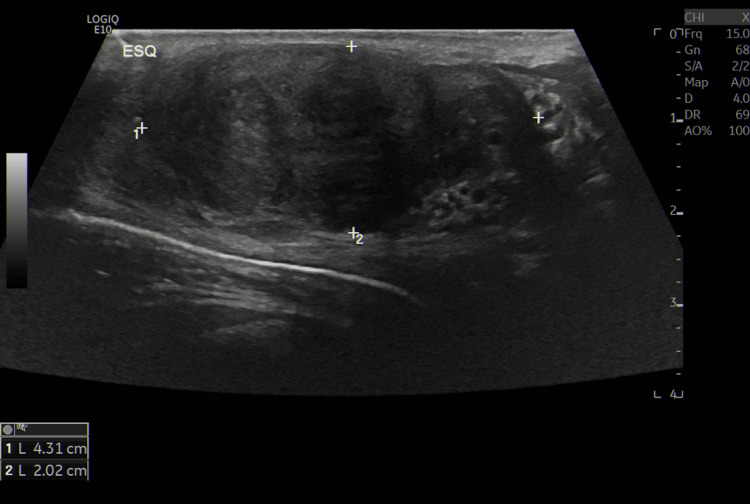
Scrotal ultrasonography-B-mode showing heterogeneous and infiltrative left paratesticular mass measuring 43 x 20 mm (longitudinal x anteroposterior)
